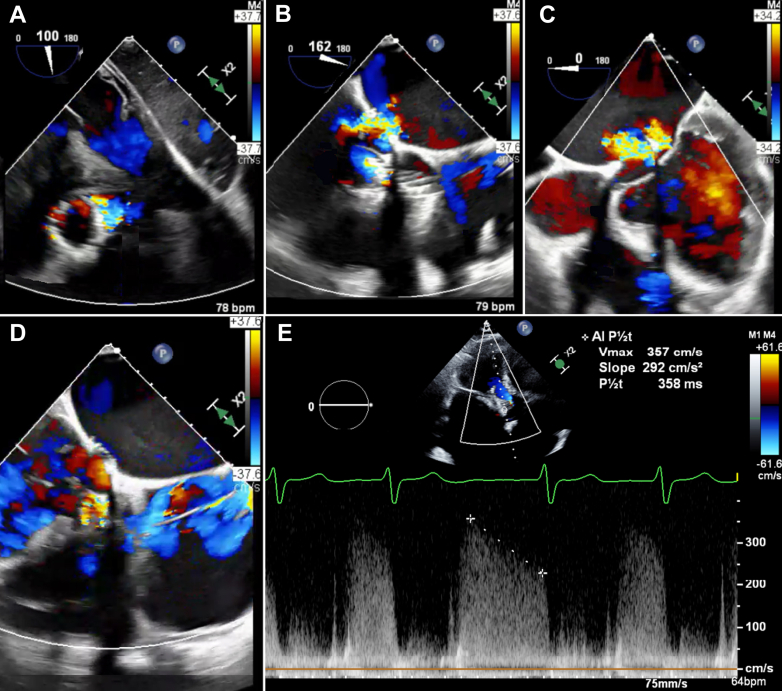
Transesophageal Echocardiogram Findings (A) Paravalvular leak (short axis). (B) Paravalvular leak (long axis). (C) Mitral regurgitation (midesophageal 4-chamber). (D) Mitral regurgitation and restriction of the anterior mitral leaflet (midesophageal reverse 4-chamber). (E) Regurgitant flow waveform (color Doppler).
